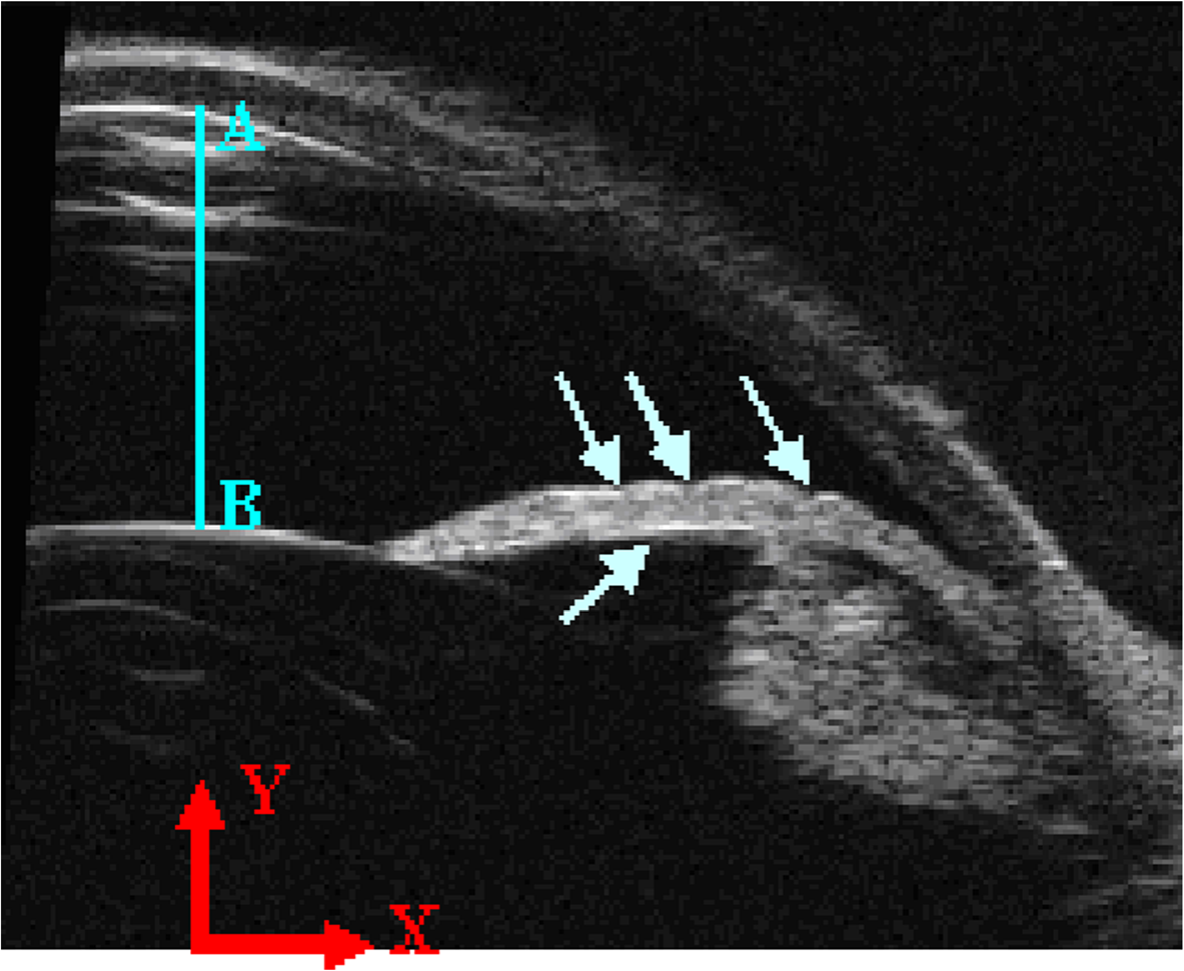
Ultrasonic image of the anterior chamber. Arrows point to the characteristic points on the surface of the iris. The anterior chamber depth AB was defined as the distance between two points whose tangent slopes were equal to zero on the edges of the inner surface of the cornea and the upper surface of the lens.
